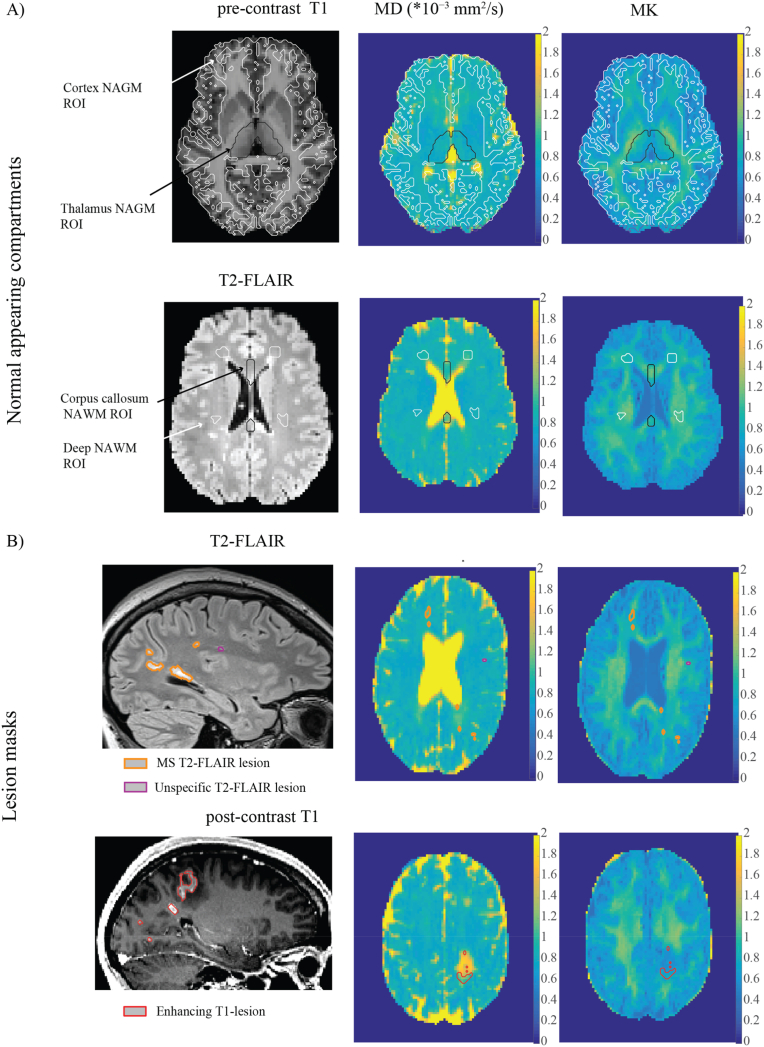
Examples of Region of Interest (ROI) placement on T1-and T2-weighted images and corresponding DKI parametric maps. Example of NAGM and NAWM ROIs on co-registered T2-FLAIR images and pre-contrast T1-images, and as overlays on MD and MK parametric maps, after co-registration and re-slicing to DKI. B) Example of lesion mask on original T2-FLAIR images and post-contrast T1-images, and as overlays on MD and MK parametric maps, after co-registration and re-slicing to the corresponding axial imaging slice in DKI.
